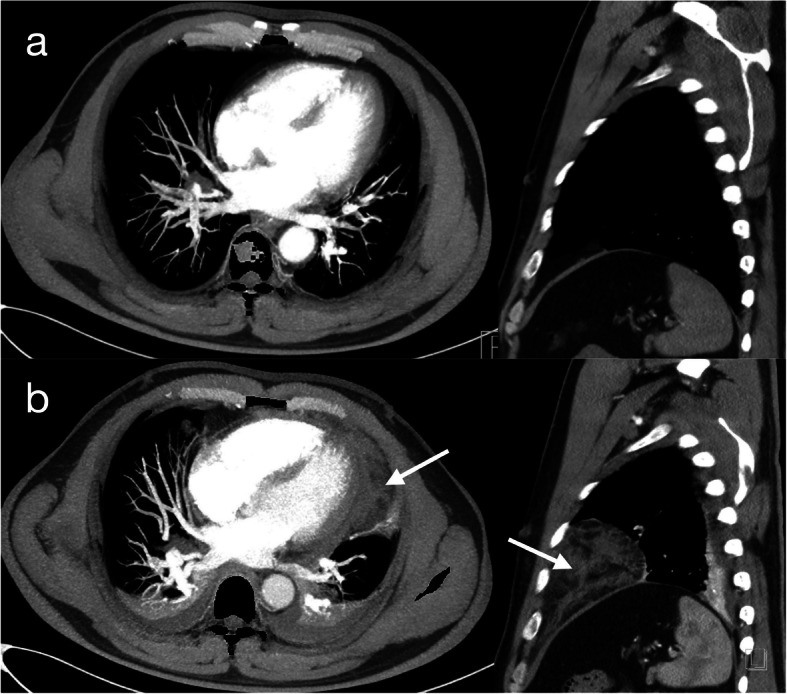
Computed tomography pulmonary angiography No mediastinal effusion occurred before the pericardiocentesis (Panel a). The white arrow shows the mediastinal effusion after the pericardiocentesis (Panel b)
